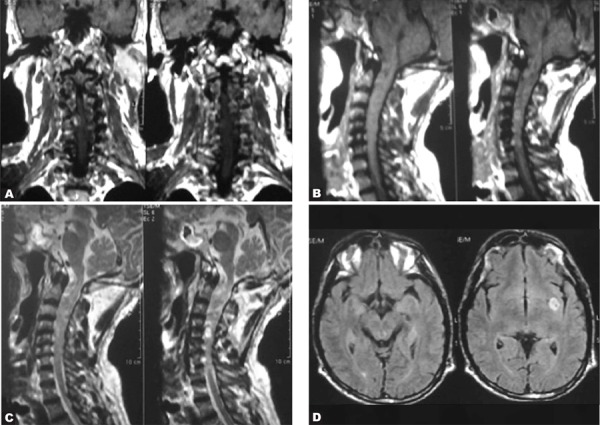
A 42-year-old man with painful skin lumps on his body and weakness in his upper limbs. A. Coronal T1-weighted MR image of the cervical cord B. Sagittal T1-weighted post contrast MR image of the cervical cord C. Sagittal T2-weighted MR image of the cervical cord D.Axial FLAIR image of the brain
